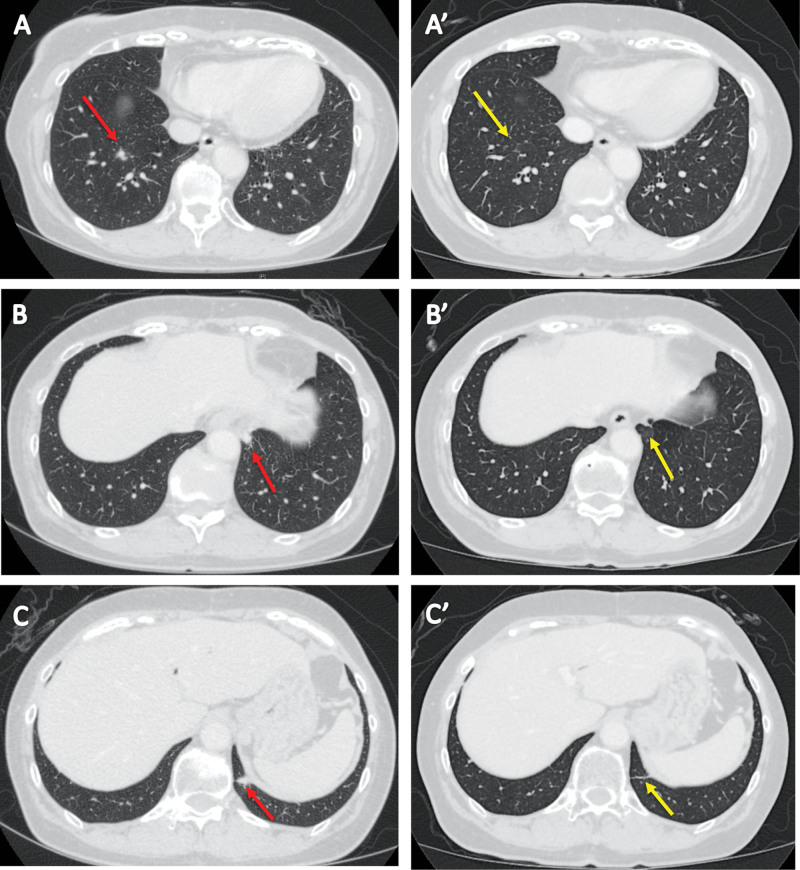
Computed tomography demonstrated multiple pulmonary nodules (red arrow), which were diagnosed as lung metastases of pancreatic cancer (A–C). These pulmonary nodules disappeared (yellow arrow) after chemotherapy (A’–C’).
